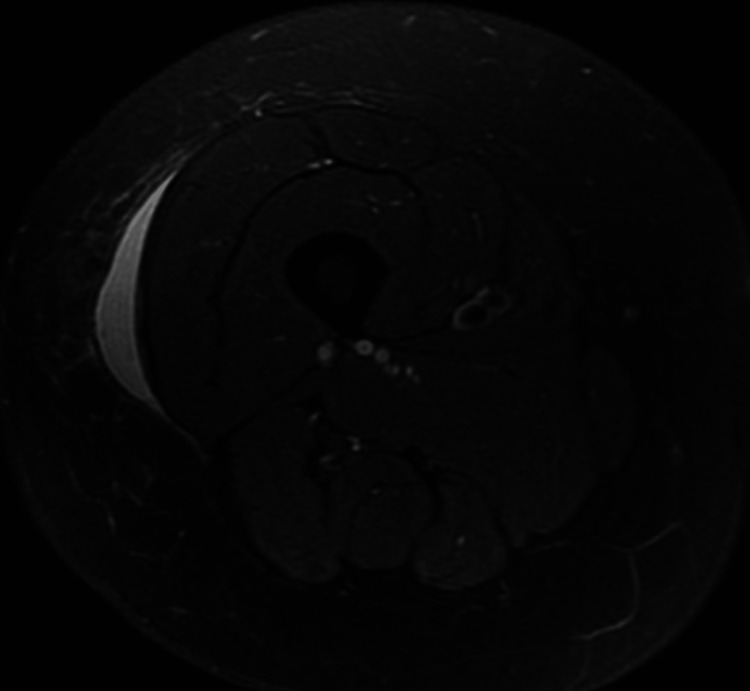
T2-weighted axial MRI through the right thigh showing a simple, hyperintense fluid collection.
